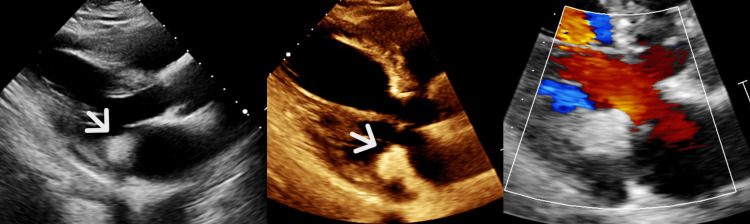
Parasternal long-axis view demonstrating an echogenic round lesion in the region of mitral annulus with mild posterior acoustic shadow (white arrow).
